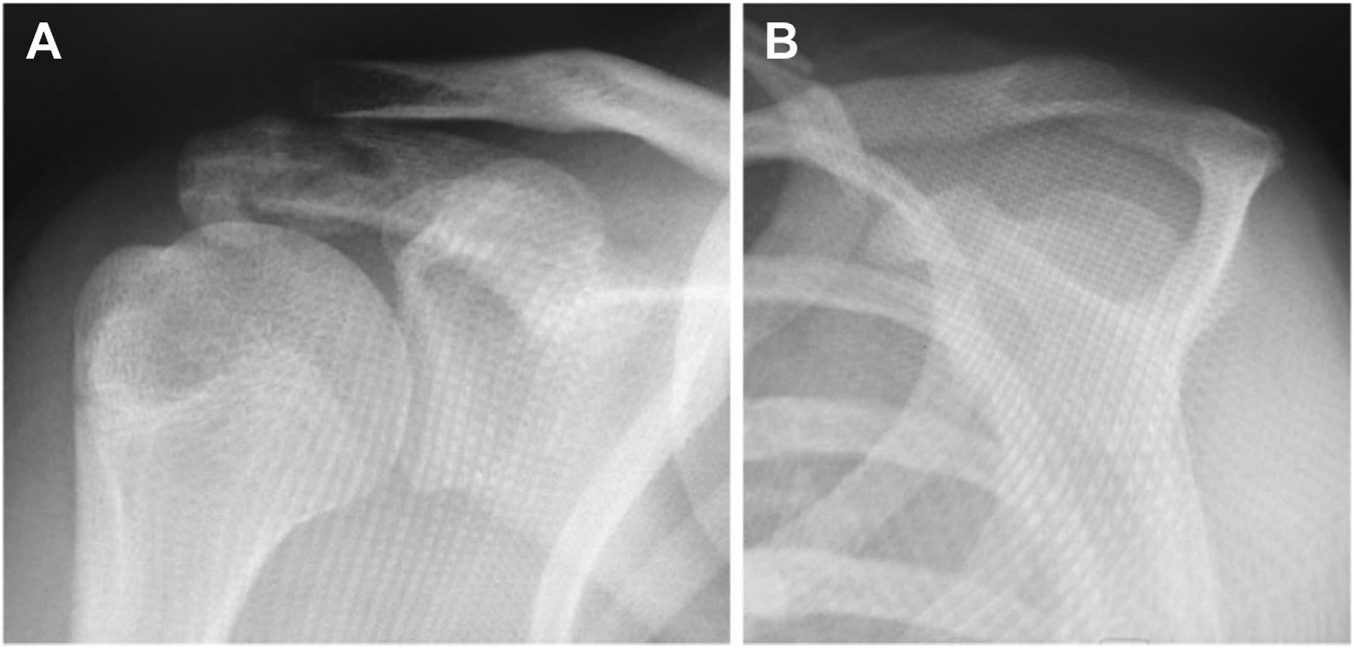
(A) Three-month postoperative shoulder anteroposterior and (B) scapular Y radiographs showing fracture healing and bone bridging between the epiphysis and metaphysis.
